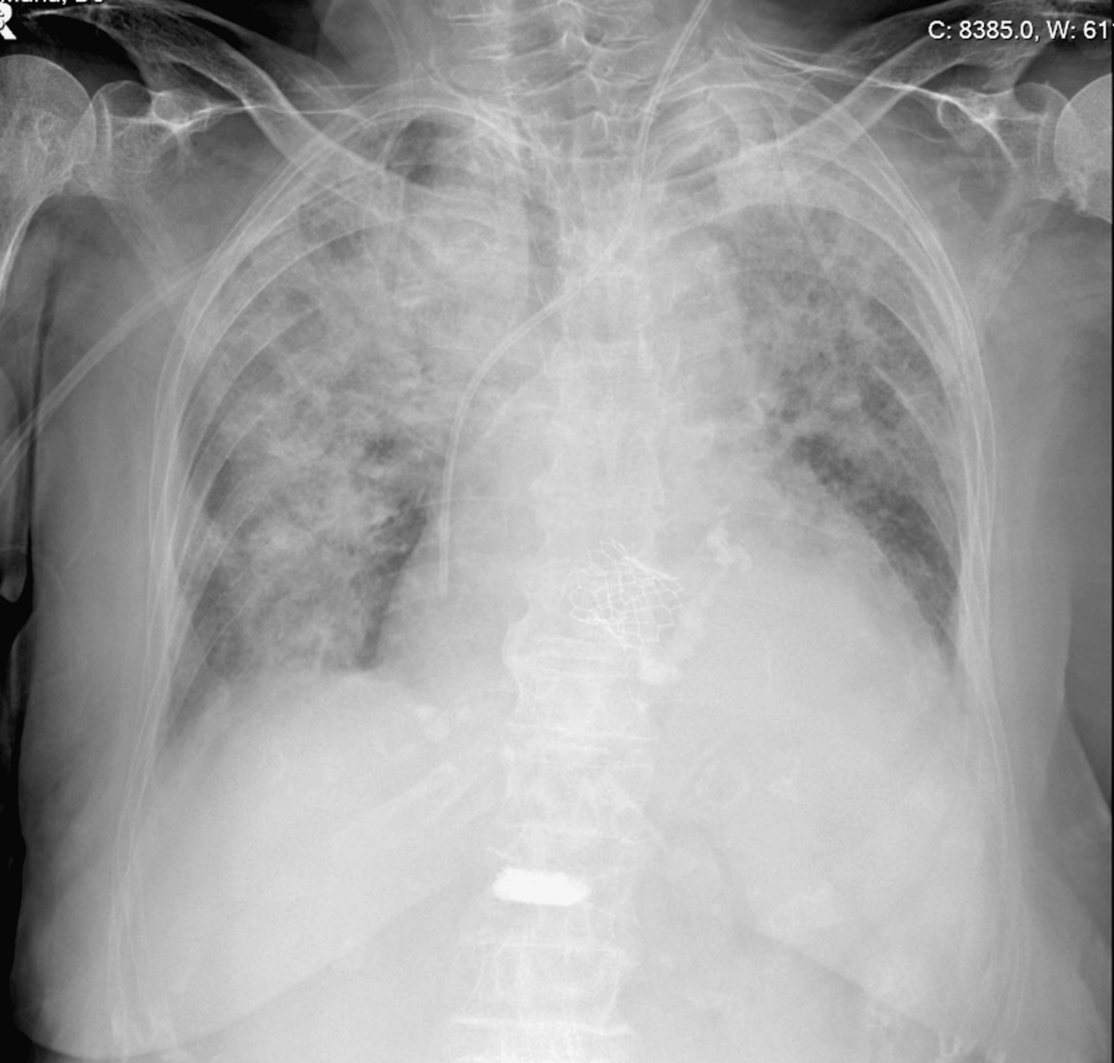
Chest radiograph showing bilateral interstitial infiltrates.
